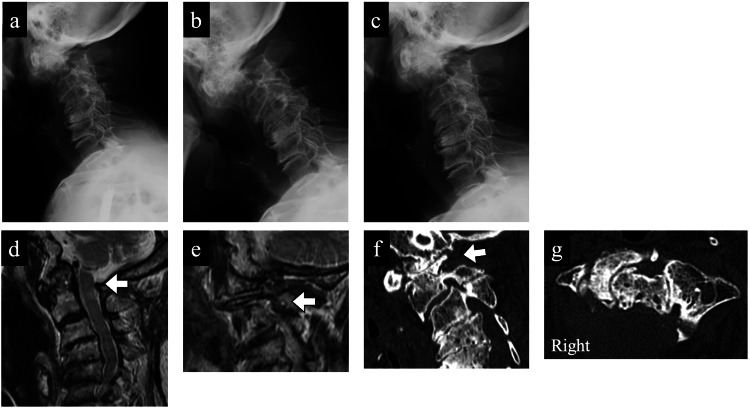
Postoperative radiological findings. (a–c) Dynamic lateral radiographs in flexion, neutral, and extension positions demonstrating no progression of atlantoaxial instability or loss of cervical motion compared to preoperative findings. (d, e) Follow-up magnetic resonance imaging (MRI) confirming sufficient decompression of the spinal cord and right C2 nerve root. (f, g) Postoperative computed tomography (CT) images showing complete removal of the bone fragments within the C1–C2 neural foramen and no evidence of recurrent stenosis or instability.
